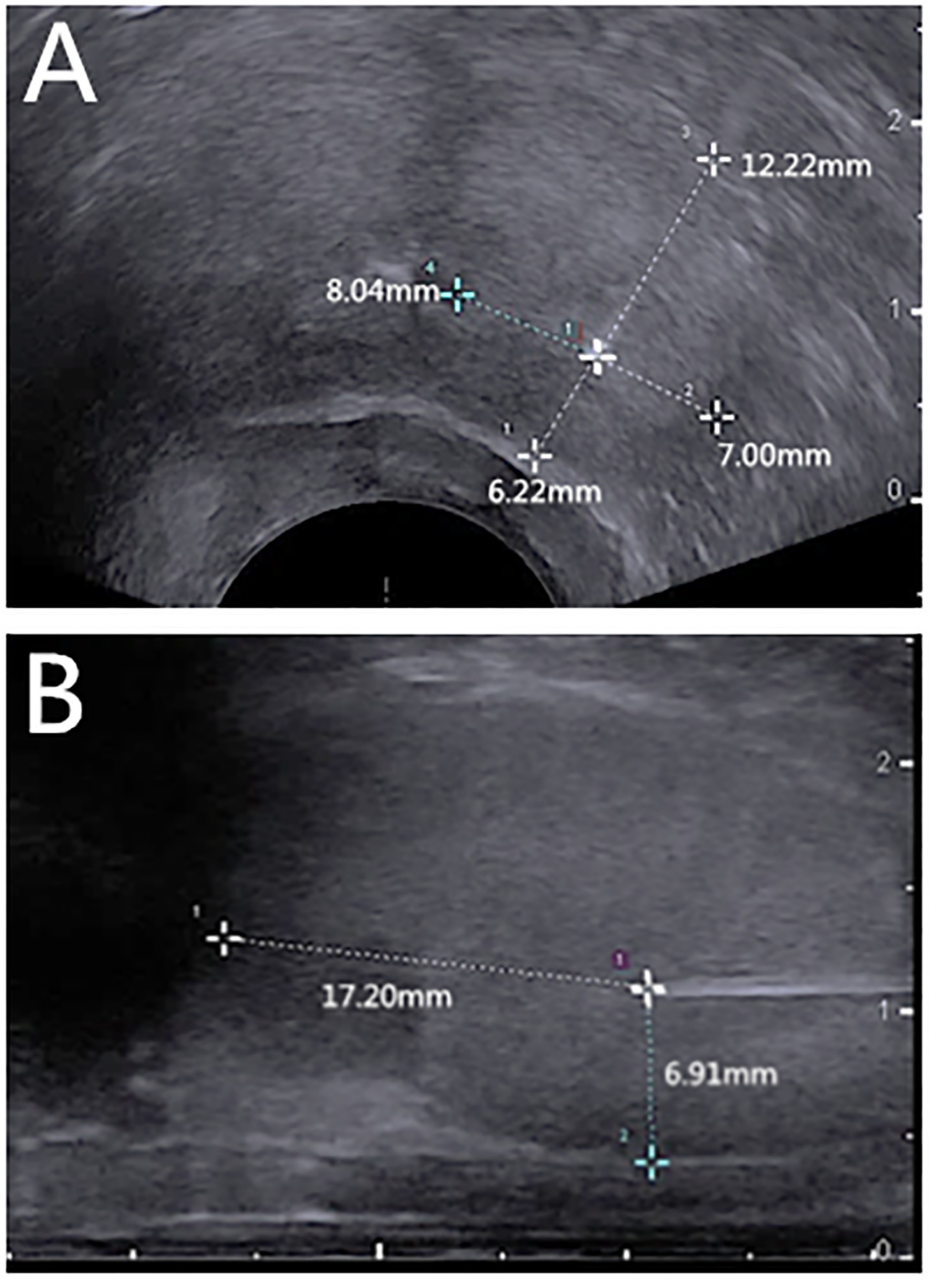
Distances from the tip of the laser fibre to the outer wall of the urethra, the rectum wall and the bladder floor on transrectal ultrasound images (A, transverse section; B, longitudinal section).
